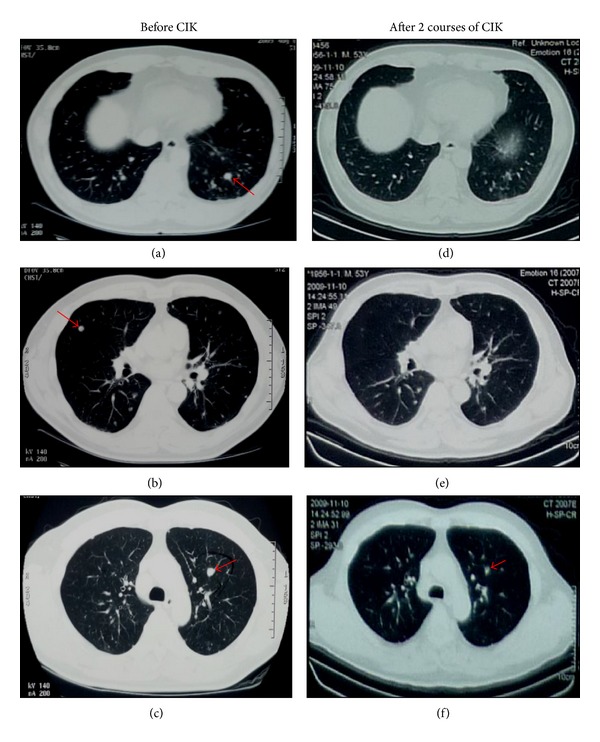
Example of chest CT before and after CIK cell treatment. ((a)–(c)) Images from Unique Patient Number (UPN) 7, who was diagnosed with pulmonary metastasis after radical nephrectomy and before CIK cell treatment. ((d)–(f)) Images from the same patient after two courses of CIK cell treatment. (a) A solid space-occupying lesion (0.9 × 1.2 cm) was observed in the left lung. (b) A circular lesion (0.5 × 0.5 cm) with high density and clear boundary was observed in the right lung. The CT value was 22 Hu in the plain scan. (c) The image shows a space-occupying lesion (1.1 × 0.9 cm) with a high density signal and a clear boundary. (d) After two courses of CIK cell treatment, the tumor burden in the inferior lobe of the left lung was significantly reduced (compared to the corresponding image in (a)). (e) Chest CT indicated almost complete absence of lesions in the left lung corresponding to the image shown in (b). (f) Shrinking of lung metastatic lesions was noted.
